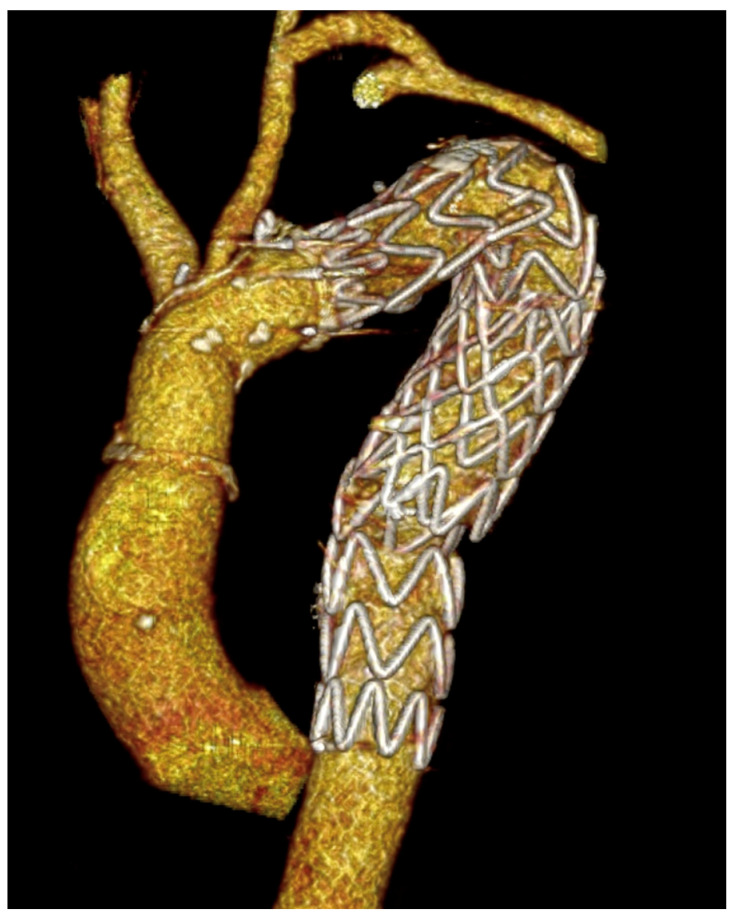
Three-dimensional volume-rendered reconstruction of the 1-year CTA.
